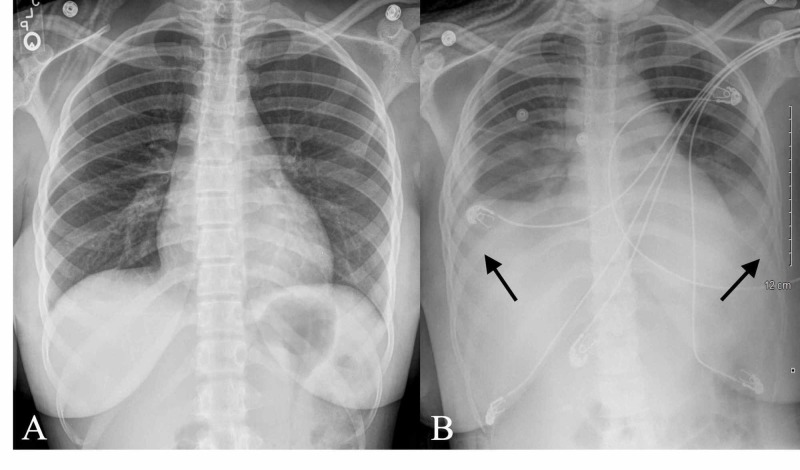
Posteroanterior and portable chest X-ray (A) Posteroanterior chest X-ray showing normal bilateral costophrenic angles and no acute intrathoracic process. (B) Portable chest X-ray on day three showing new bilateral lung base opacities. Arrows showing new bilateral pleural effusions.
